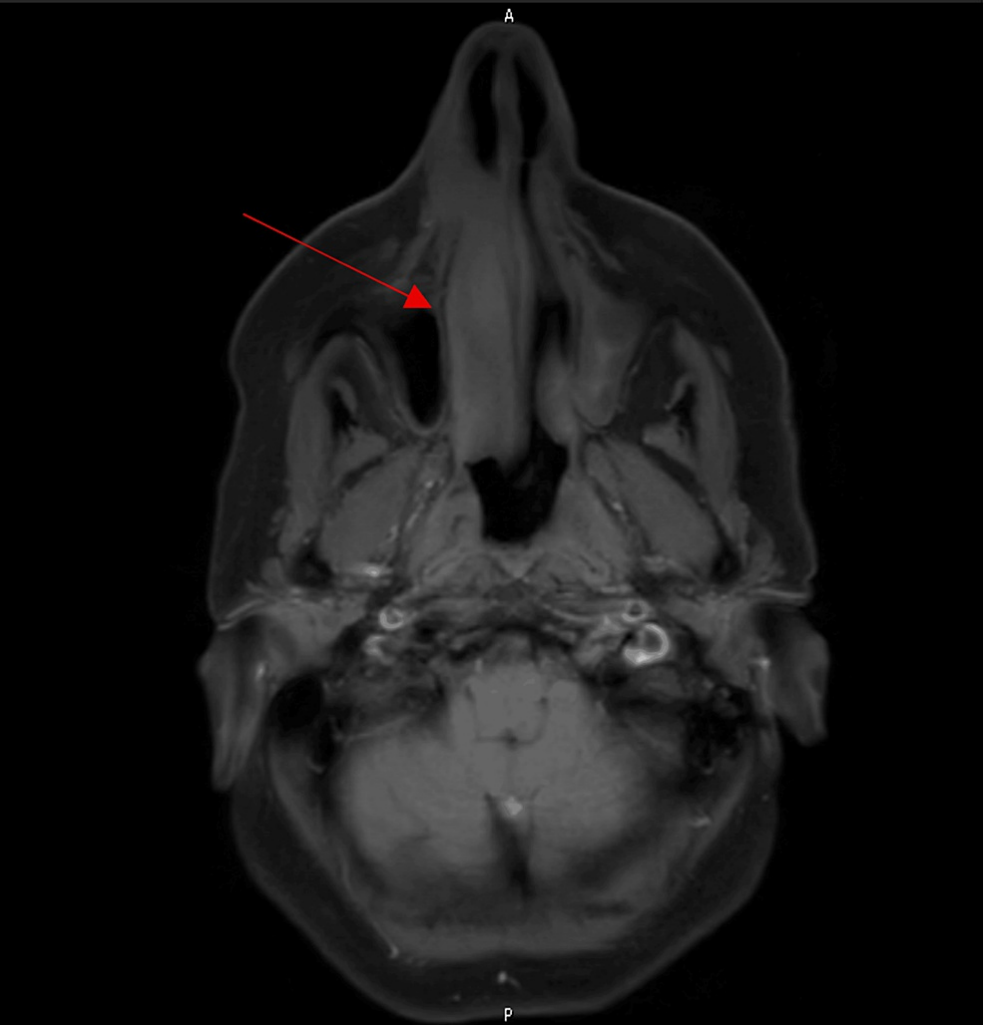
MRI facial showing a soft tissue mass in the nasal cavity
